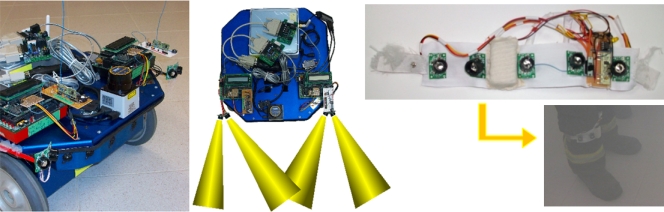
Erratic Videre mobile platform equipped with 2 measurement boards based on TDoA (left). Ultrasonic beam patterns (center). Sonar elastic ring, suitable to be attached to a person leg (right).
